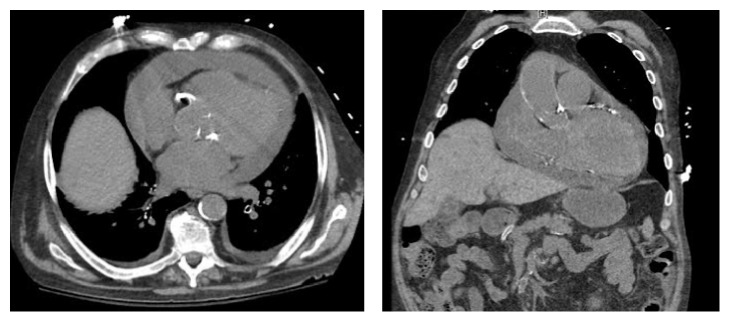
CT imaging demonstrated large pericardial effusion.
